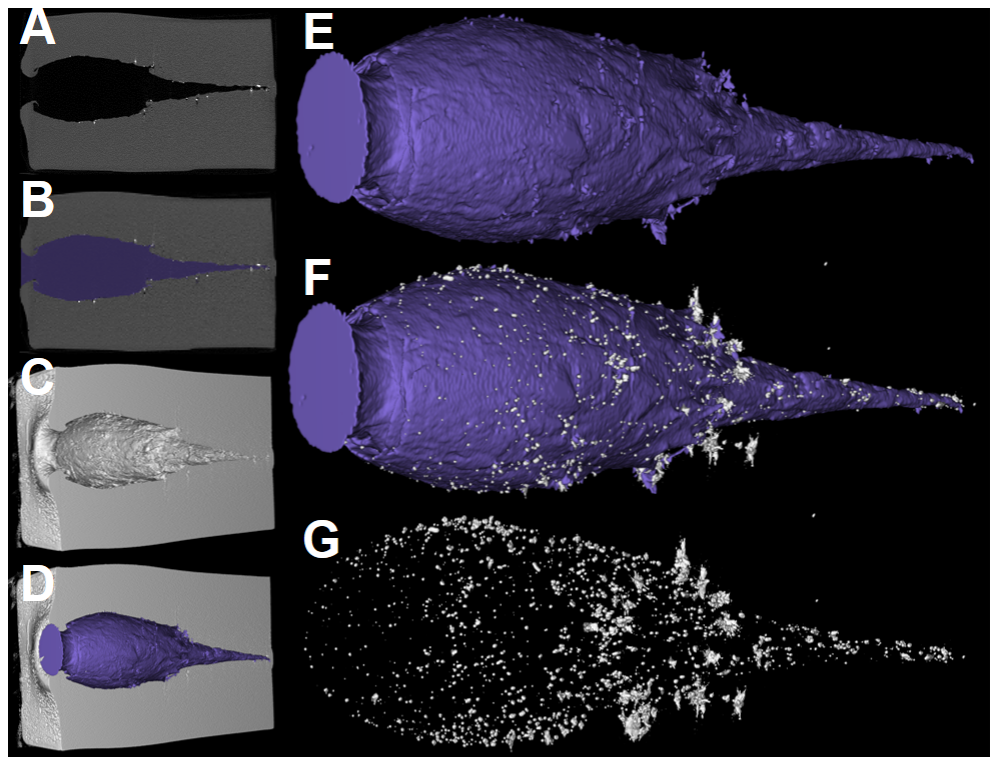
CT method. (A) CT slice of the soap block with a cavity. Air outside the block and in the cavity appears black. The soap appears gray and the metal fragments as small white spots. (B) Segmented cavity shown in blue. (C, D) 3D renderings of the virtually cut soap block and the segmented cavity. (E) 3D rendering of the cavity. (F) Cavity with lead fragments. (G) Cloud of lead fragments.
